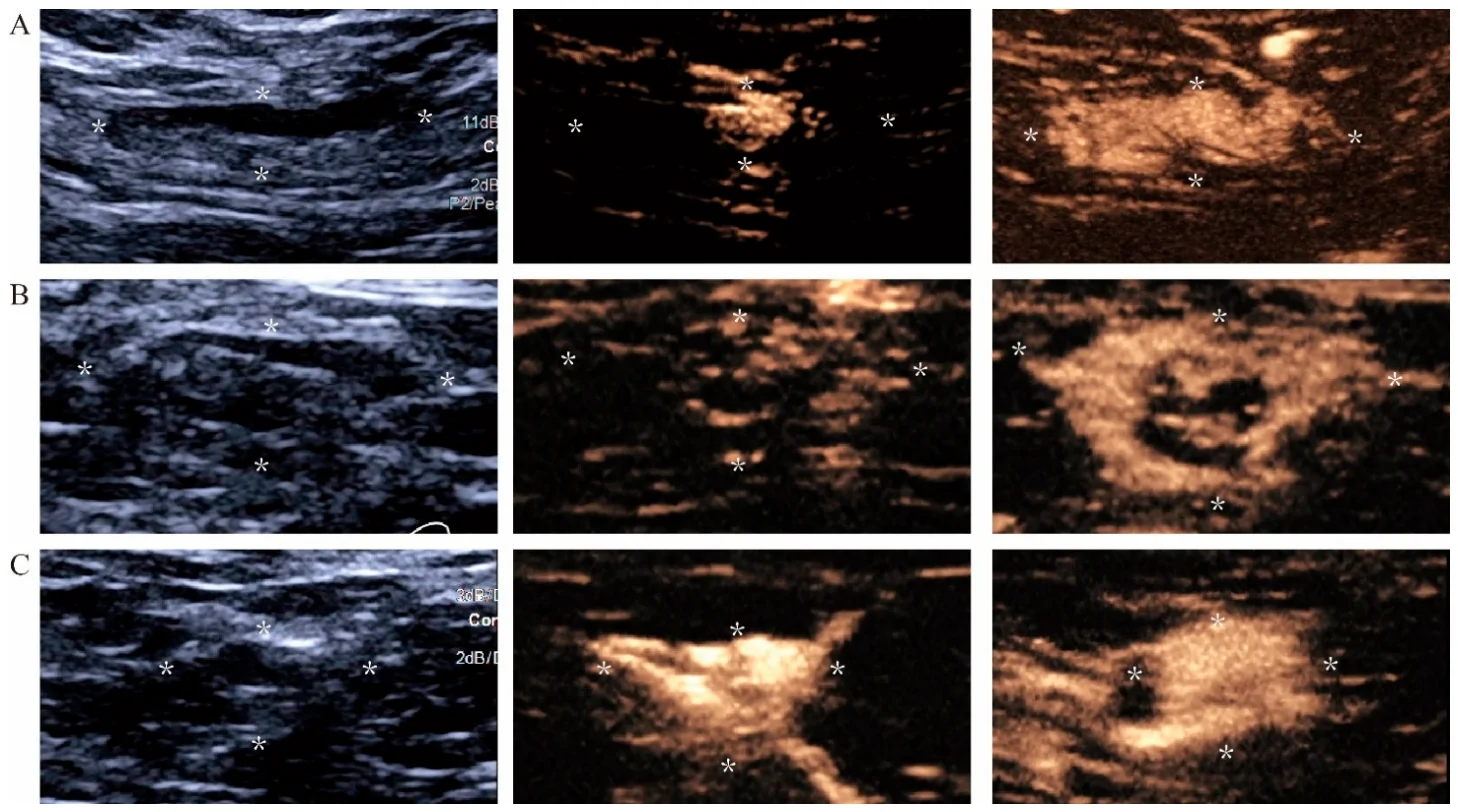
Comparison of SLN images among patients with CEUS-negative/SLNB-negative findings (A), CEUS-negative/SLNB-positive findings (B) and true-negative status (C). Left: B-mode ultrasonography; Middle: PCEUS; Right: ICEUS. SLN, sentinel lymph node; CEUS, contrast-enhanced ultrasound; SLNB, sentinel lymph node biopsy.
